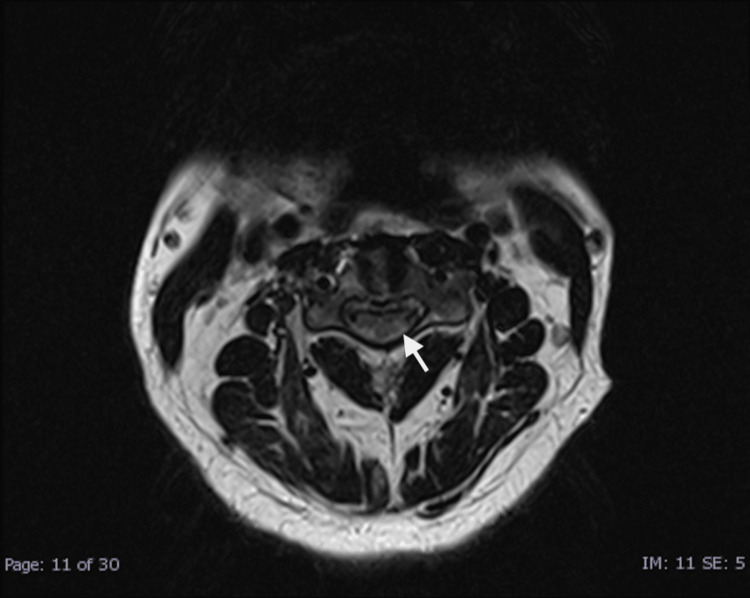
Axial T2-Weighted Image Axial T2-Weighted MR image of the spinal cord shows hyperintense signal change, indicative of ischemic spinal cord sprinkling and white cord syndrome.
